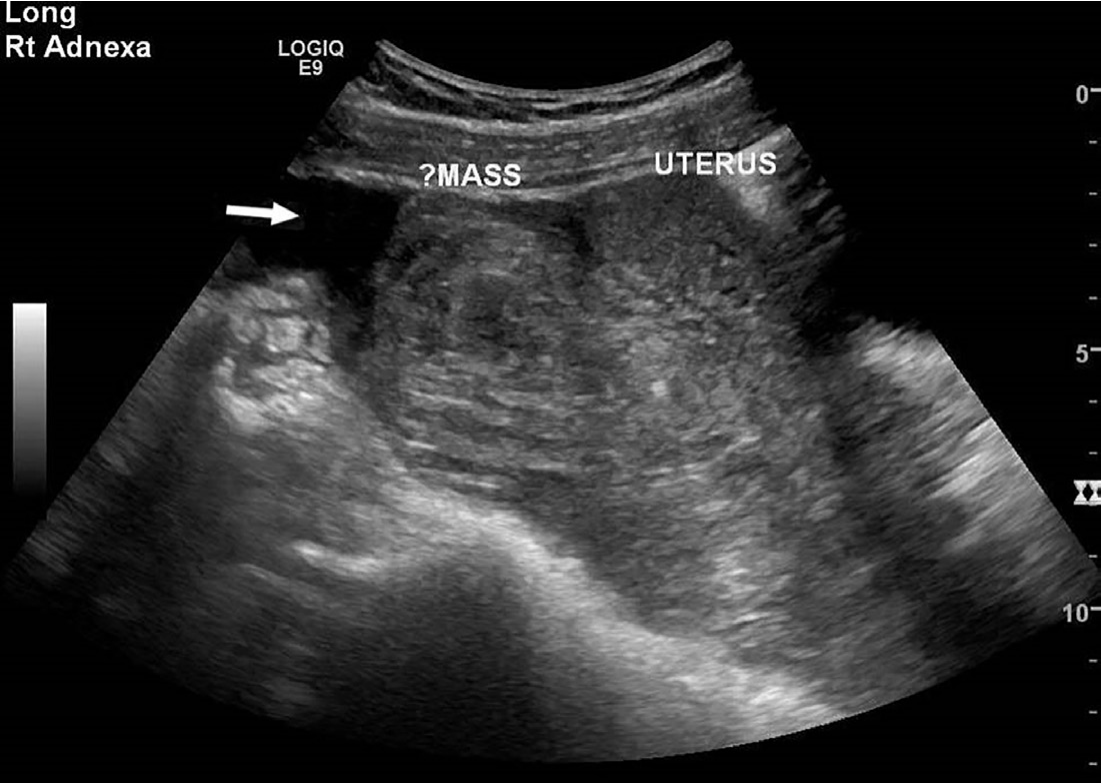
Short axis. Transvaginal ultrasound demonstrating a 10-centimeter heterogeneous mass posterior to the uterus, moderate free fluid in the pelvis (arrow), and no evidence of intrauterine pregnancy or adnexal abnormality.
